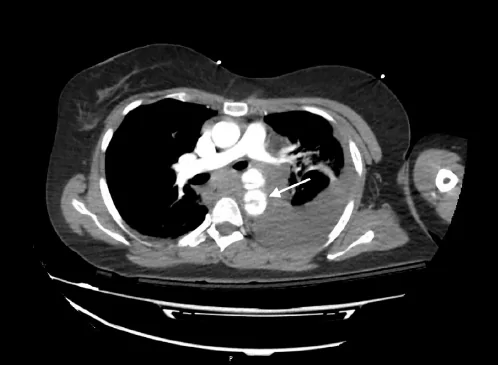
Computed tomography of the chest with contrast in axial view demonstrating transection of the aortic wall (arrow).
